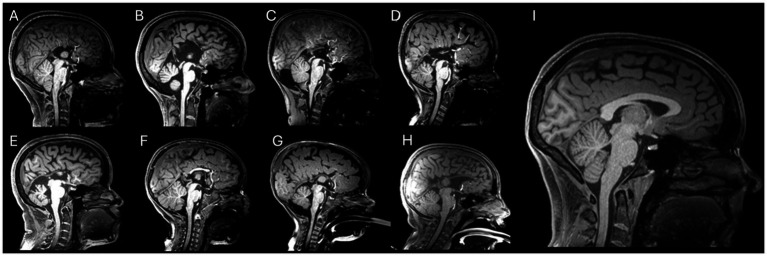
Mid-sagittal T1-weighted anatomy. CCD participants (A–H) and neurotypical control (I). Subtypes: callosal agenesis (A–E,G,H); callosal hypoplasia (F). All images are central mid-sagittal slices displayed with identical field-of-view and window/level. This panel provides anatomical context for the within-subject comparisons across diffusion sampling schemes.
